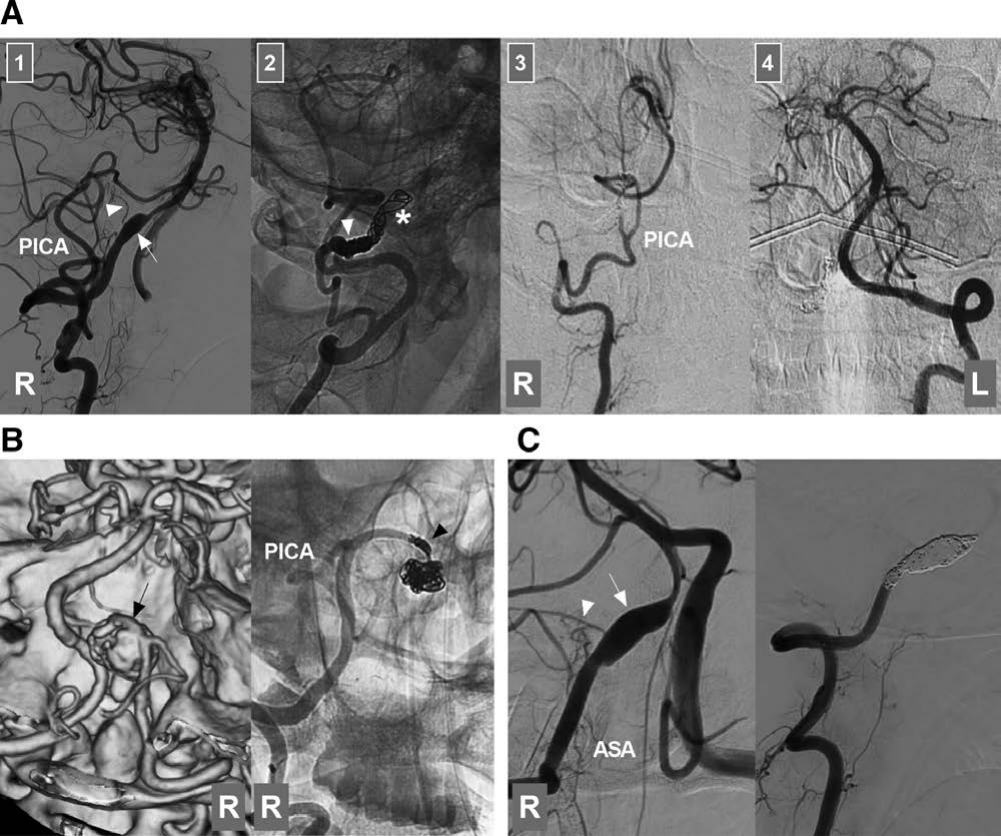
Deconstructive treatment for intracranial VA dissecting aneurysms without PICA involvement. (A) Proximal VA PAO: panel 1: DSA image showing a right VA dissecting aneurysm (arrow) and a perforating artery (arrowhead) emerging from the aneurysm; panel 2: Unsubtracted DSA image showing that the aneurysm (asterisk) is packed loosely and the proximal VA (arrowhead) is densely coiled; panels 3 and 4: 6-month follow-up DSA images of the bilateral VAs showing that the aneurysm has been cured, the right VA is supplying only the PICA, and the left VA is supplying the BA. The patient did not experience new neurologic deficits during and after treatment. (B) Trapping plus PAO: left panel: Computed tomography angiography showing a right VA dissecting aneurysm (arrow); right panel: DSA image showing trapping of the aneurysm and occlusion of the proximal VA (arrowhead). (C) Trapping with ischemia symptoms: left panel: DSA image showing a right VA dissecting aneurysm (arrow), a perforating artery (arrowhead) emerging from the aneurysm, and the ASA emerging from the end of the aneurysm; right panel, DSA image showing coiling of the aneurysm and occlusion of the perforating artery and ASA; postoperatively, the patient experienced symptoms of brainstem ischemia and recovered gradually but incompletely. ASA = anterior spinal artery, DSA = digital subtraction angiography, L = left, PAO = parent artery occlusion, PICA = posterior inferior cerebellar artery, R = right, VA = vertebral artery.
